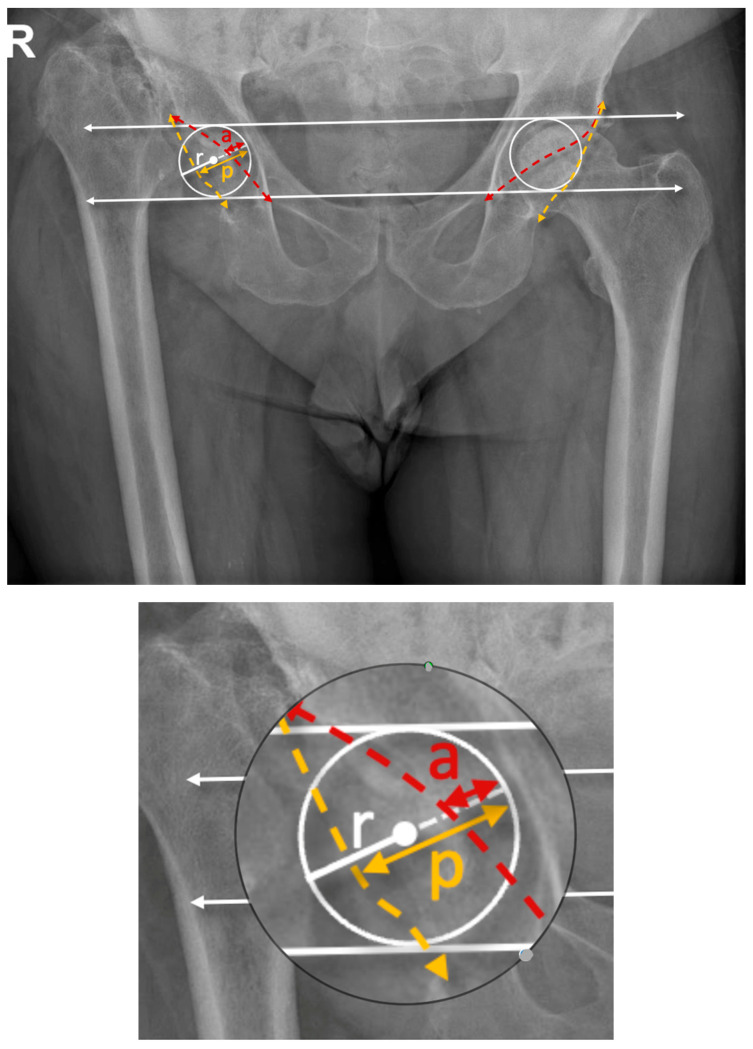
A proposed methodology for anterior wall index measurements in cases with femoral head destruction/amputation (modified after Siebenrock et al. [11]) on a pelvis AP radiograph. The proposed measurement method by our team involves placing a circle at the level of the acetabulum, connecting three anatomical landmarks: the acetabular roof (rim and dome), the medial wall, and a tangent line passing through the acetabular tear drop. The radius (r) and diameter of the circle are afterwards determined. The anterior wall projection (a, red continuous line) is measured along the circle diameter from the medial wall to the intersection of the anterior wall with the circle diameter, while the posterior wall projection (p, yellow continuous line) is measured from the medial wall to the intersection of the posterior wall with the diameter. The anterior wall index (AWI) is calculated as a/r, and the posterior wall index (PWI) as p/r. (red, interrupted line—anterior acetabular wall projection; yellow interrupted line—posterior acetabular wall projection); upper white arrow—acetabular roof, lower white arrow—line connecting radiological teardrops.
